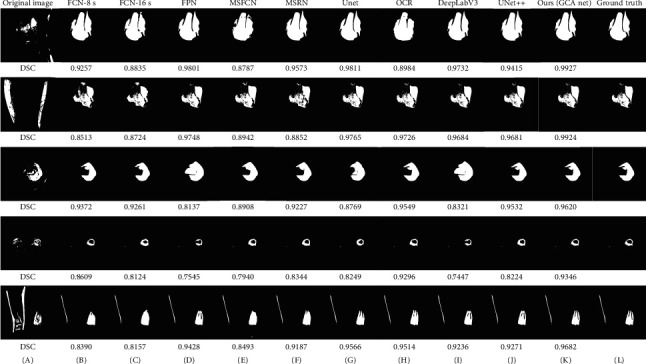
The segmentation effects of models on some osteosarcoma MRI images.
